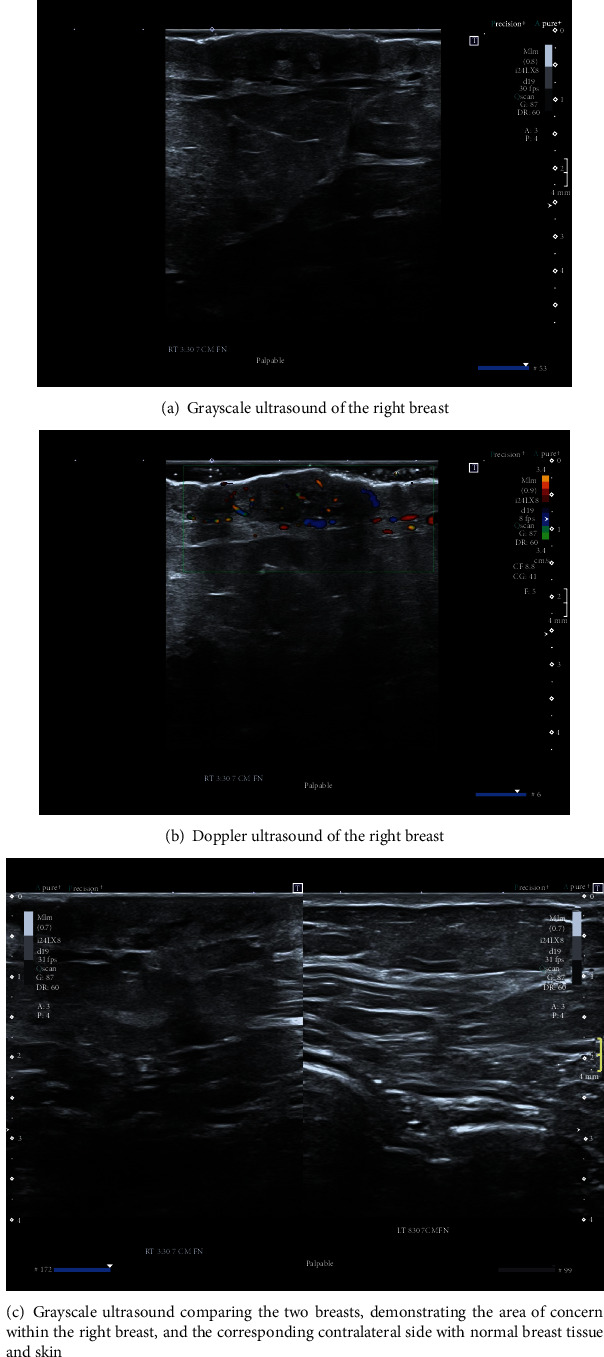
(a–c) Selected ultrasound exam on the right breast shows marked skin thickening measuring up to 7.2 mm, in the area of concern (a), associated with increased flow (b).
